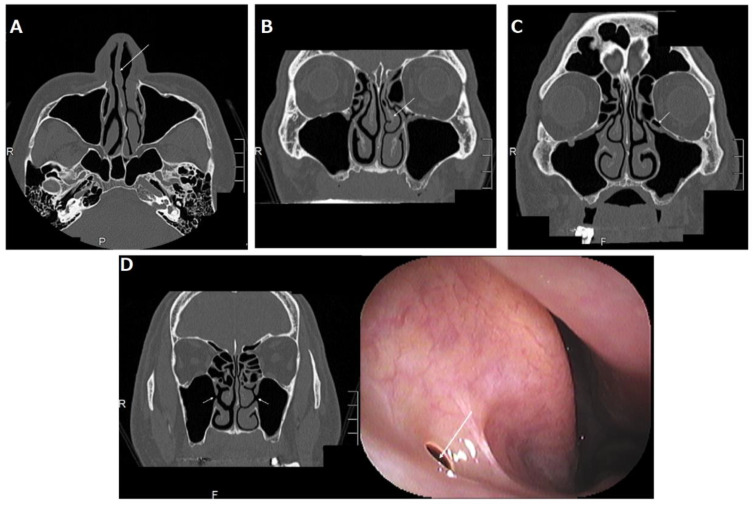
(A) Right nasal septal deviation (arrow); (B) left paradoxical middle turbinate (arrow) and left concha bullosa of the middle turbinate; (C) left Haller cell (arrow); (D) bilateral accessory ostium of the maxillary sinus (arrow in CT (left panel)) and right accessory ostium (arrow in nasal endoscopic examination (right panel)). This was originally published in *Case Reports in Otolaryngology*: Suzuki-Yamazaki, M.; Takahashi, K.; Takada, S.; Kato, Y.; Baba, Y. “A successful treatment regimen for the prevention of sinusitis after maxillary sinus floor elevation surgery in a high-risk case.” *Case Rep. Otolaryngol*. 2020, 2020, 6869805 [16].
